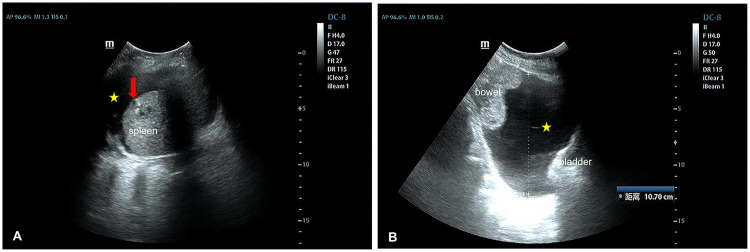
Abdominal ultrasonography reveals (A) discontinuity of the splenic capsule contour at the diaphragmatic surface with heterogeneous parenchymal echotexture (red arrow), and a significant perisplenic anechoic fluid collection (yellow star); (B) massive anechoic fluid accumulation in the lower abdominal cavity (yellow star).
